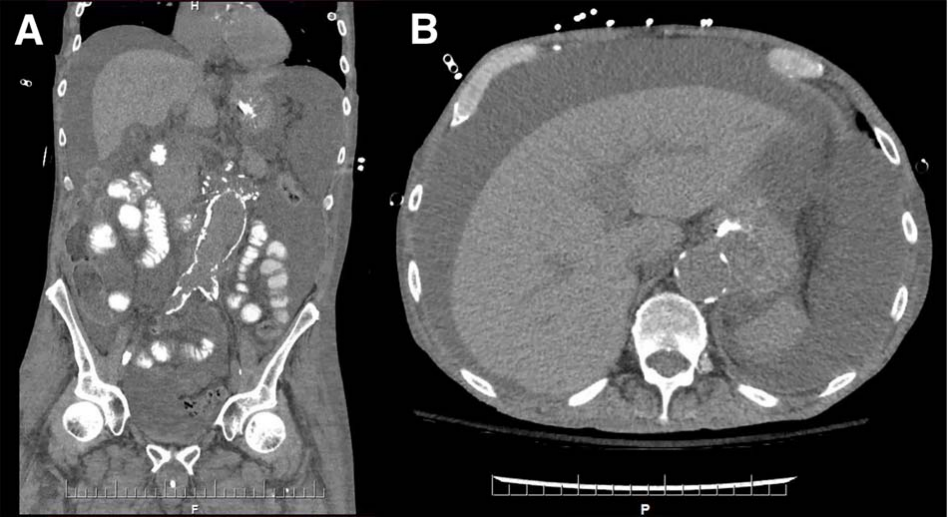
Coronal (A) and axial (B) sections of non-contrast enhanced abdominal computed tomography showing accumulation of large volume of peritoneal fluid. Aortic graft appears intact.
